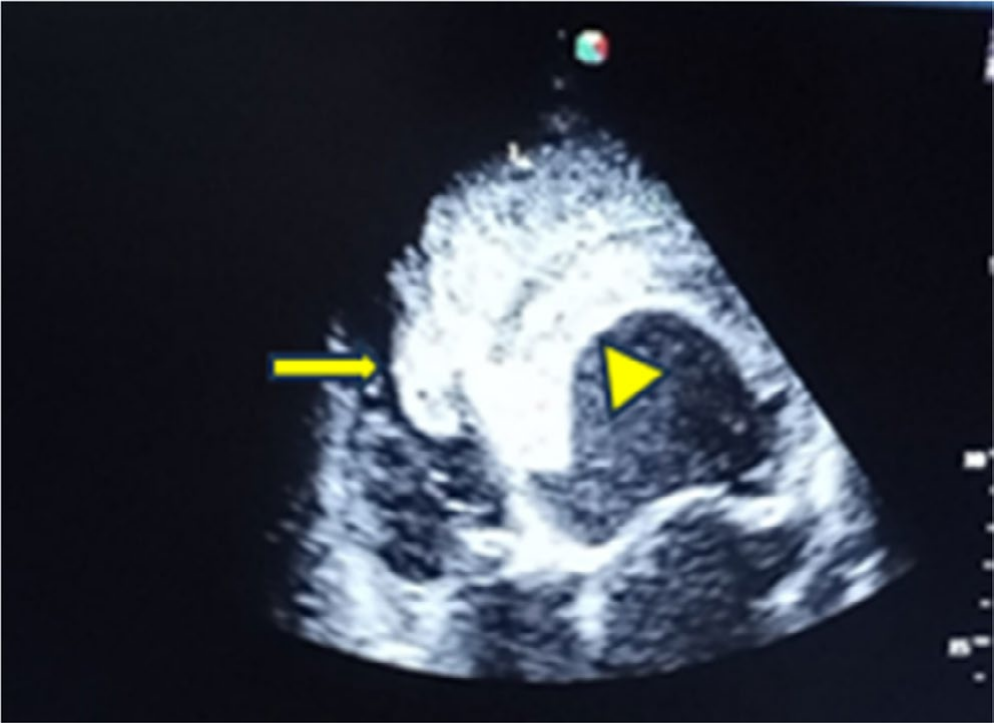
A 2‐dimensional echocardiographic picture showing a large left ventricular thrombus (arrowhead) and a solitary laminar right ventricular thrombus (arrow).
